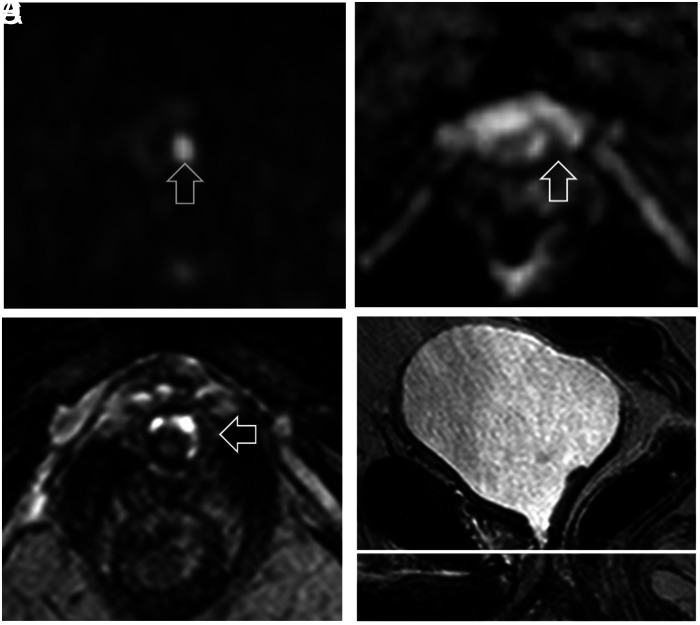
PI-RRADS category 3 based on biparametric MRI, 5 years after RP for prostate adenocarcinoma Gleason 7 score, in 87-year-old patient with PSA increased by 0.67 ng/mL. The lesion appears hyperintense on DWI at high *b* value (arrow in A), moderately hypointense on ADC map (arrow in B) and slightly hypointense on T2W images (arrow in C). The lesion is located at perianostomosis in the left at 2-4 clock cranially 10 mm above a through plane passing through the lower margin of the pubic symphysis (arrow in D).
